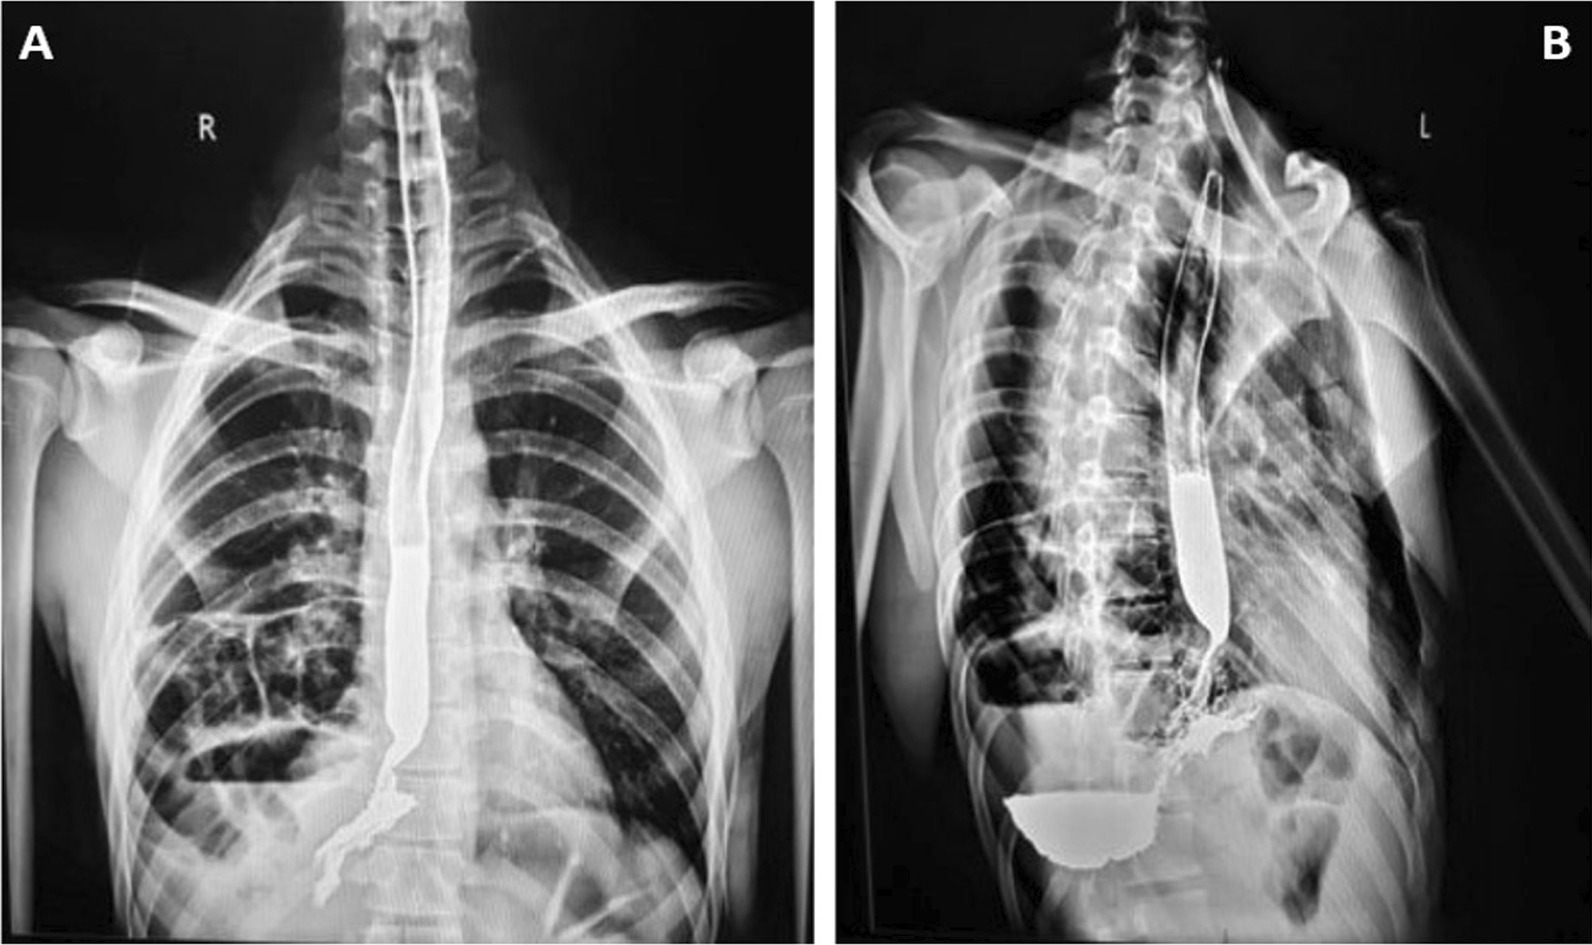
Barium test visualized the actual position of the stomach lying above the liver. A The front contrast radiograph showed the stomach lying on the right side of chest with normal opacification of esophagus and a cavitating lesion on the right side below the lung. B The lateral view of contrast radiograph confirmed the finding visualizing the stomach filled with contrast agent at the distilled end
